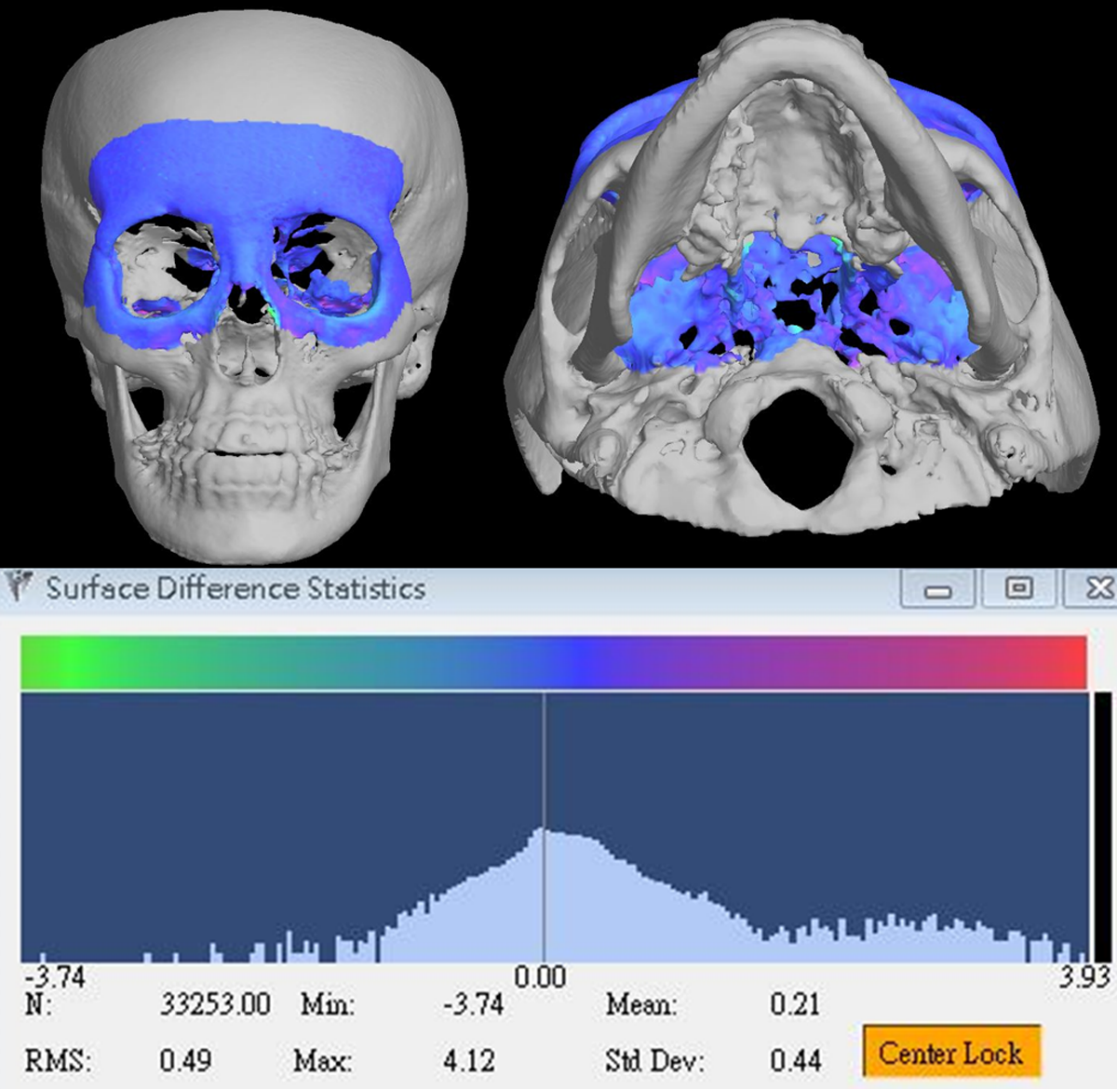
Accuracy of the cranial base registration in the color map of distance between the registered pre- and postoperative CBCT images (case no. 21).
